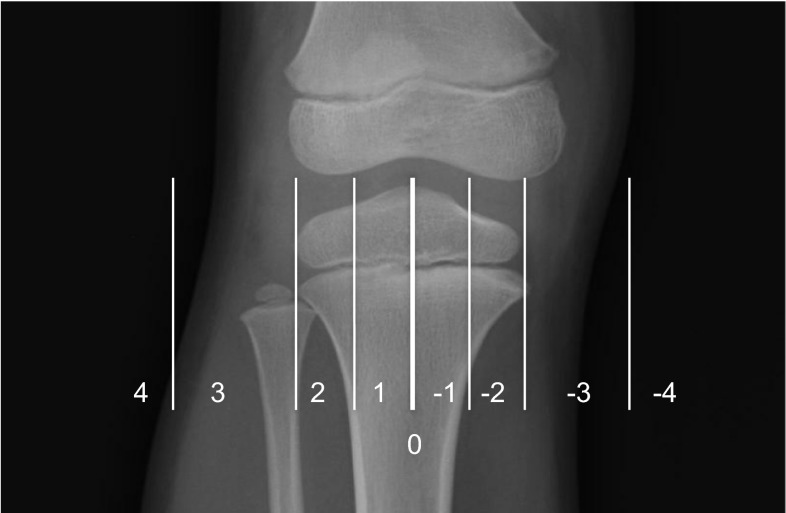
Radiograph showing the zones of the knee. Zone 0 is a* vertical line* through the central point of the tibial plateau (*thick*
